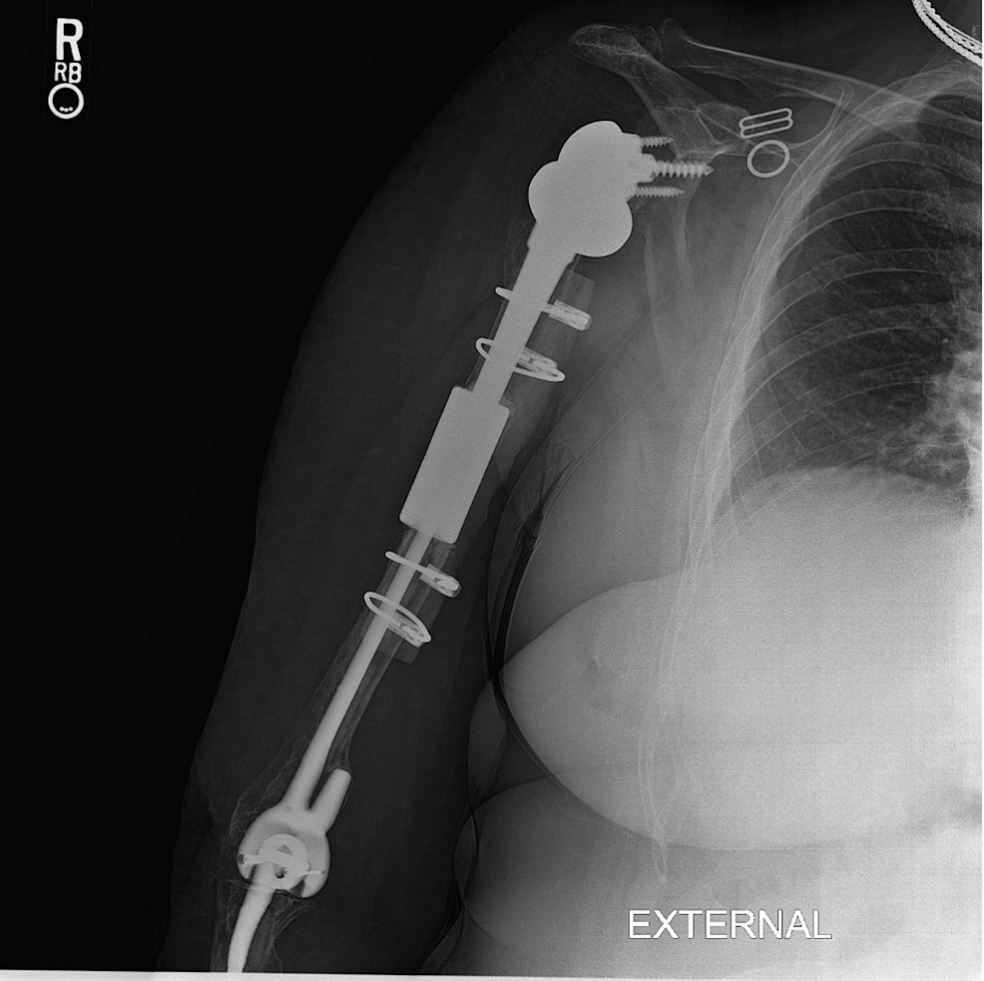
External rotation radiograph of the right humerus at six-month follow-up.
